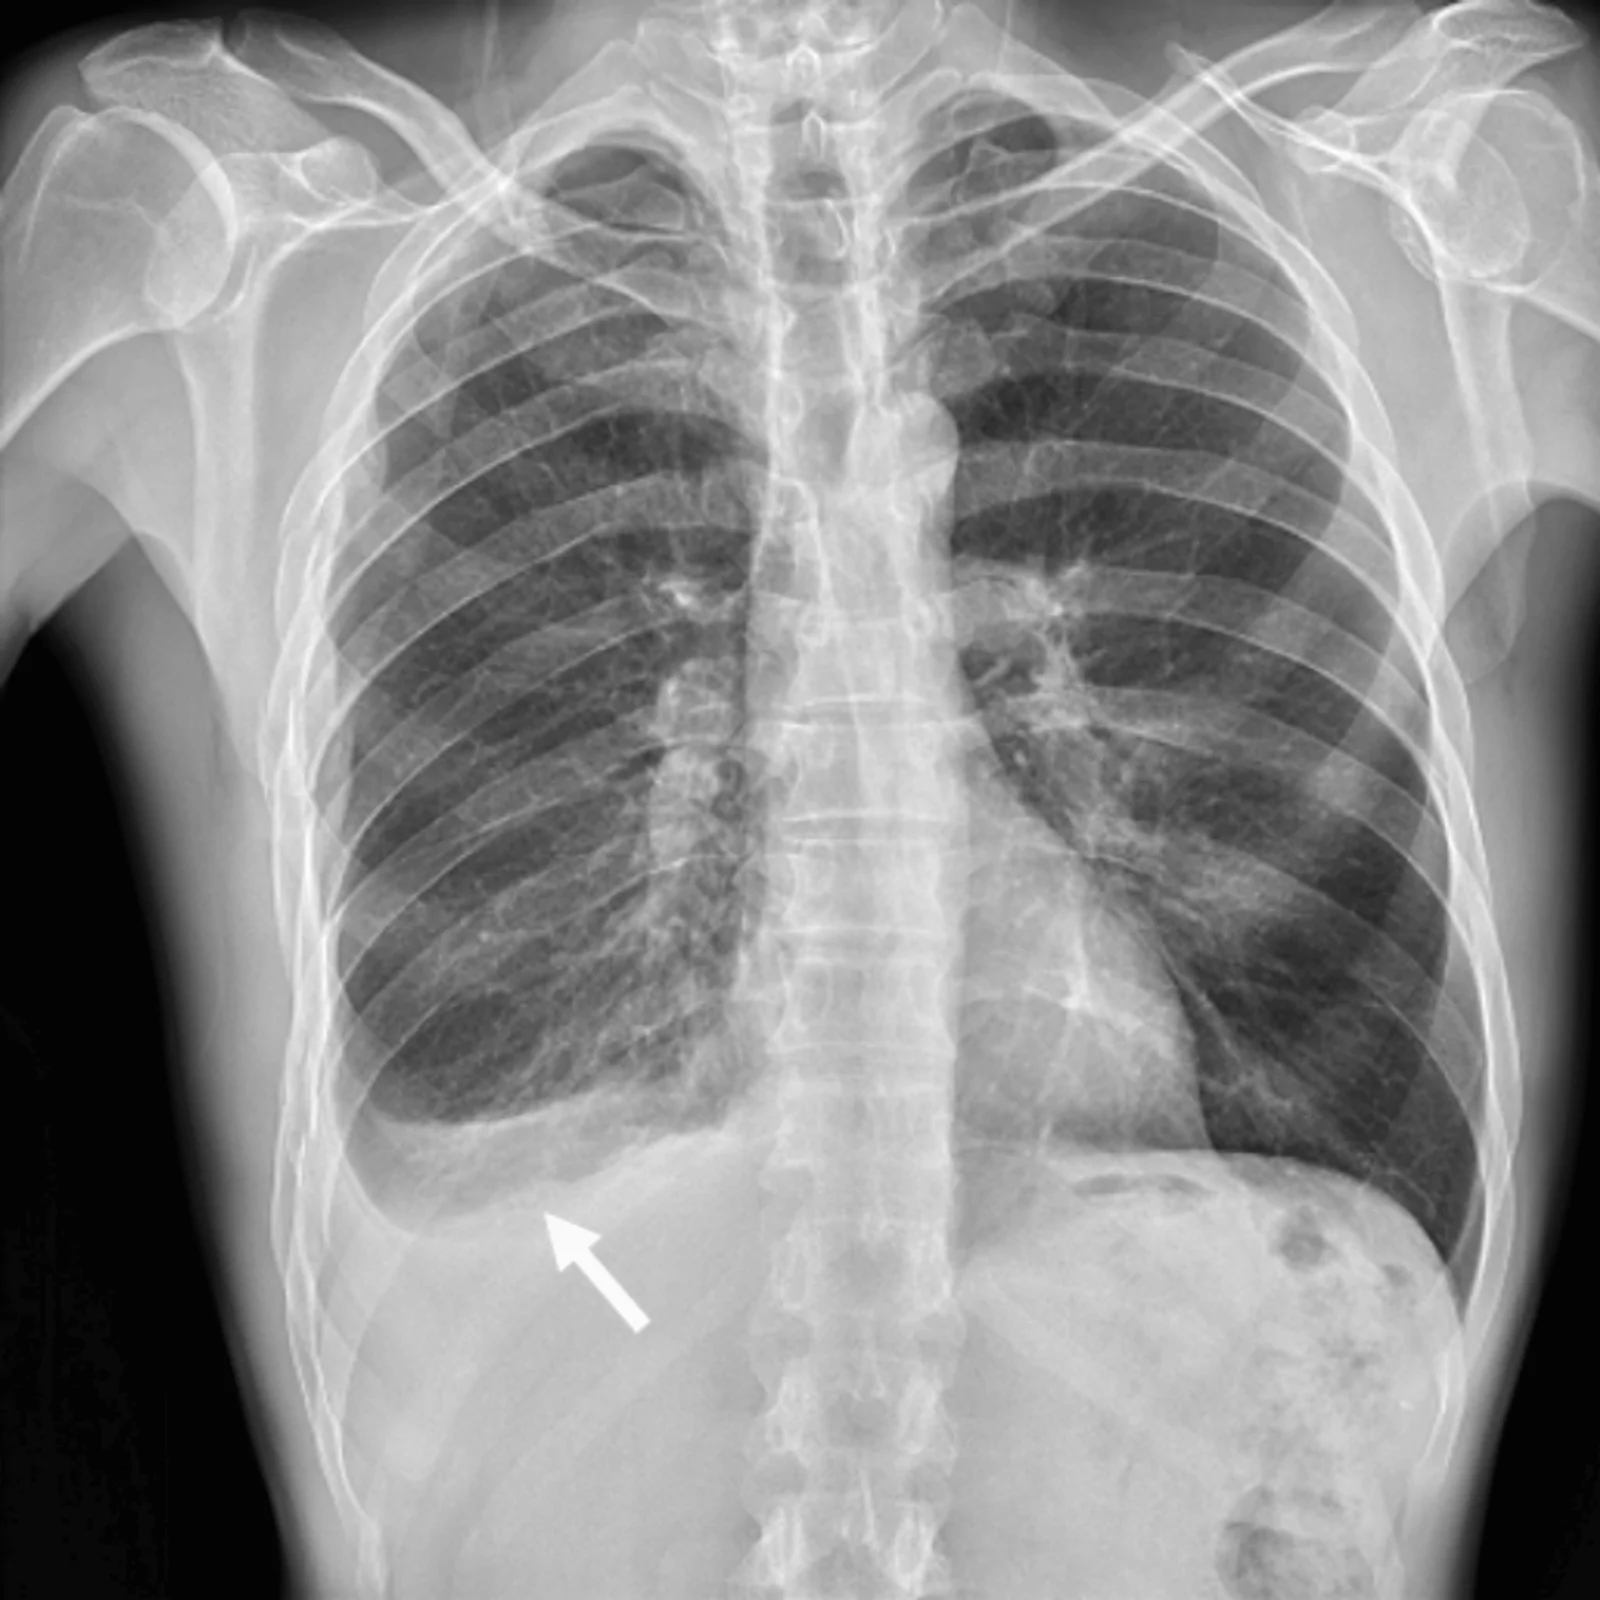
Final pre-discharge anteroposterior chest radiograph on day seven of admission. An upright chest radiograph obtained prior to hospital discharge demonstrates stable findings. Compared to previous radiographs, there is residual right-sided pleural thickening and trace fluid at the right lung base (white arrow) with blunting of the right costophrenic angle, and no evidence of recurrent hemopneumothorax.
